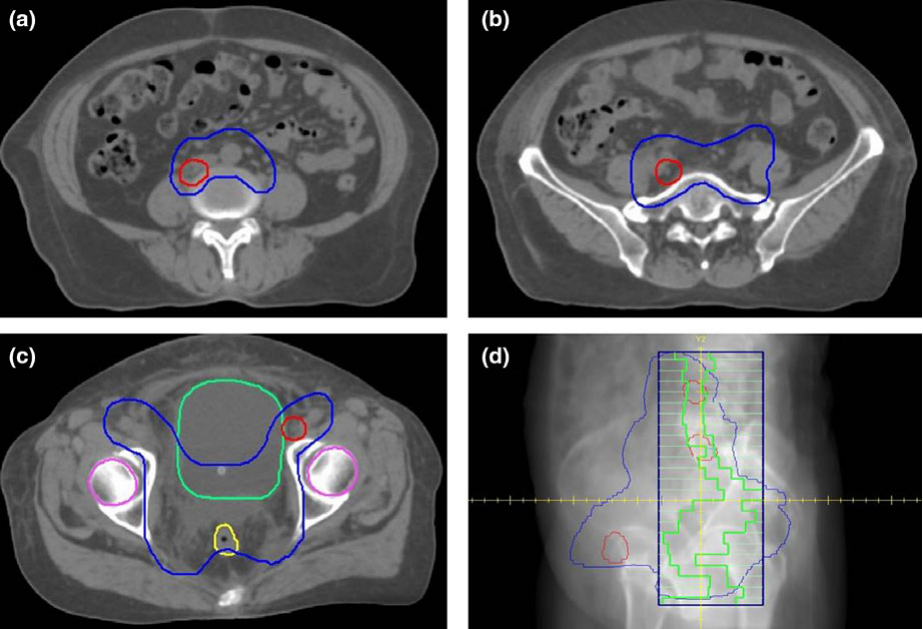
The shapes of the planning target volumes (a–c) and a typical segment (d) for a cervical volumetric modulated arc therapy plan. The red contour denotes the PTV60 and the blue contour denotes the PTV45 in (a), (b), and (c). d: Digitally reconstructed radiography for a typical segment from the beam's eye view (gantry = 112°). The green outline represents the shape of the segment.
